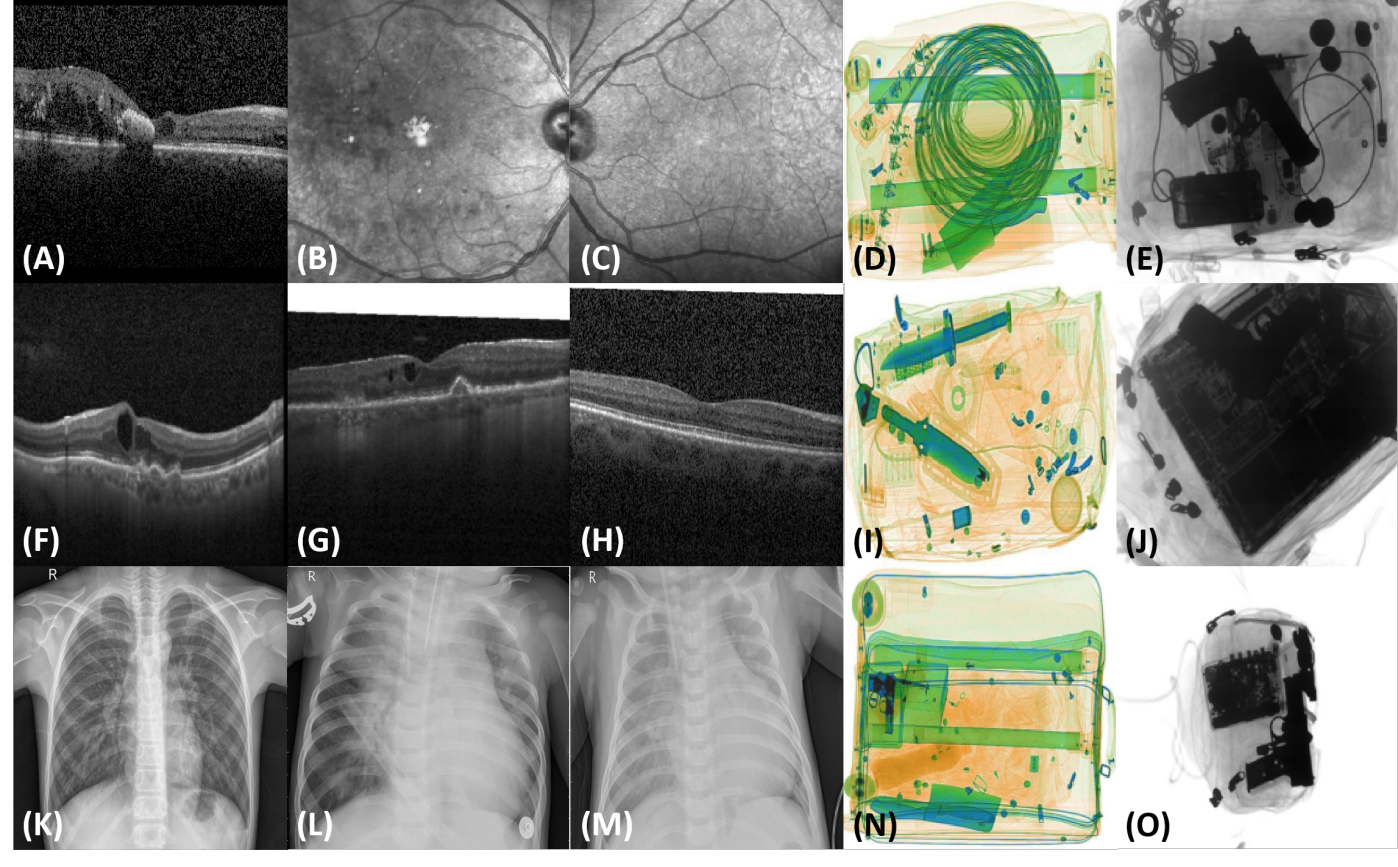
Example images showcasing different classification tasks. (A,F–H) show retinal OCT images of DME, wet AMD, dry AMD, and a healthy subject, respectively. (B,C) show retinal fundus scans of DME and a healthy subject, respectively. (K–M) show chest X-ray scans of pneumonic patents, and the rest of the scans showcase the examples of suspicious items in colored (D,I,N) and grayscale (E,J,O) X-ray scans.
